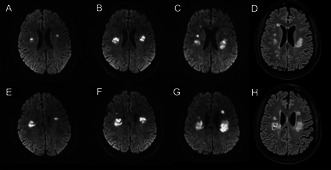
FIGURE 1 MRI Brain diffusion‐weighted sequences, axial sections in patient 1 demonstrated restricted diffusion within the bilateral corona radiata and centrum semi‐ovale (A, E).
